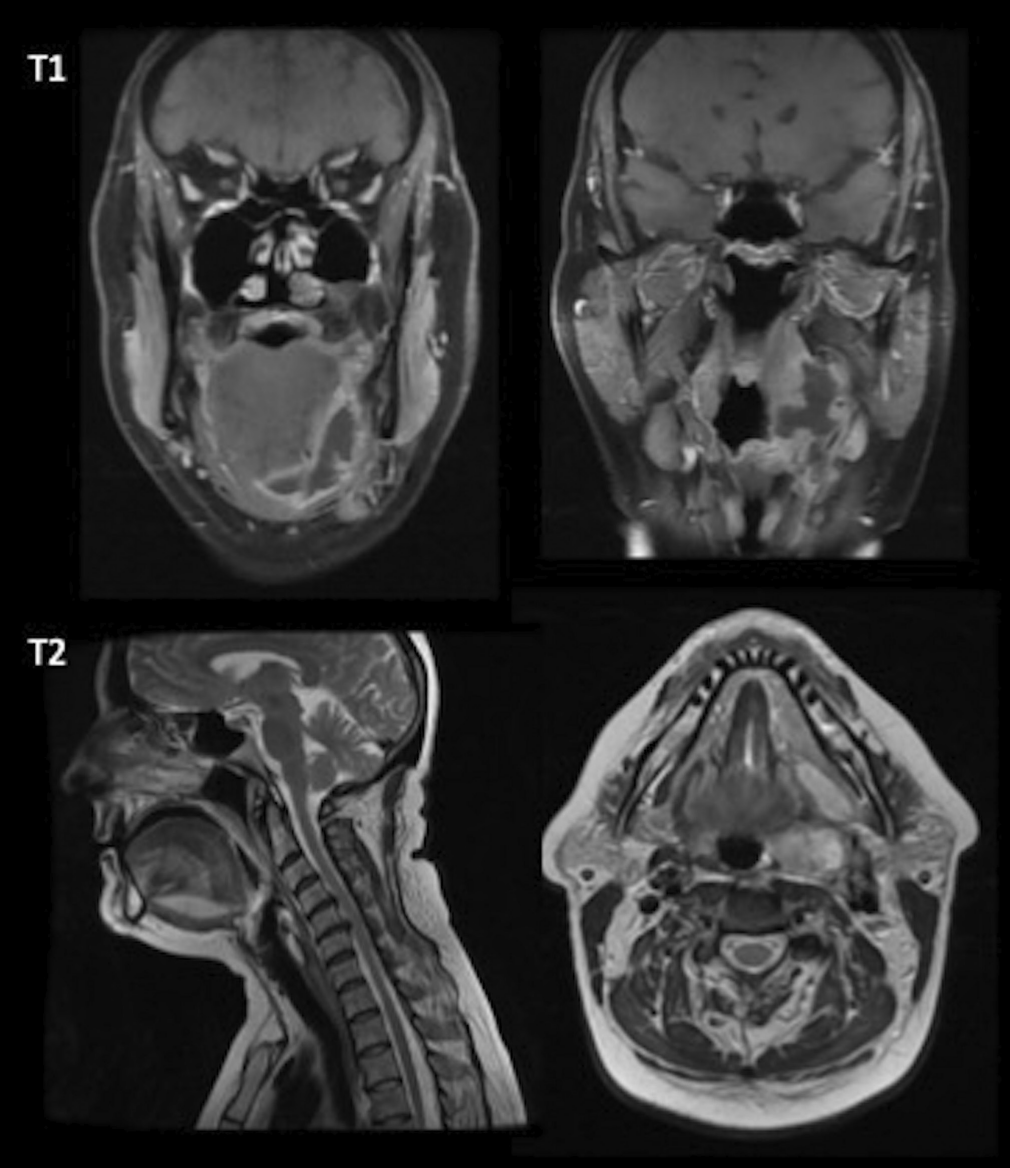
Magnetic resonance imaging (MRI) demonstrating an inflammatory mass lesion and multiloculated collection centered on the left palatine tonsil, which extends to the left lateral and anterior floor‐of‐mouth.
